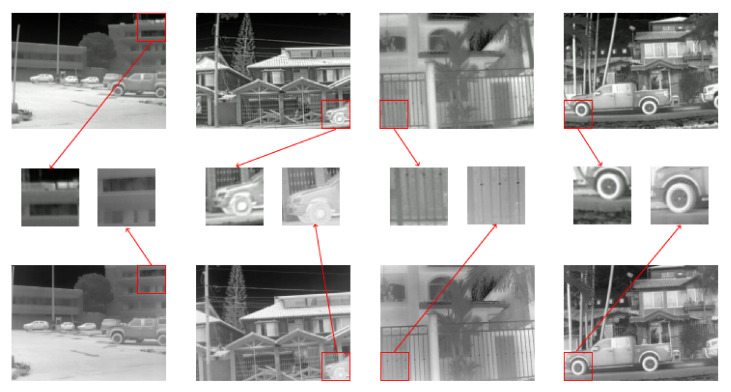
Examples of thermal images from [16]: (top) MR images from Axis Q2901-E (320 × 240), used in the current work as LR images; (bottom) HR images from FC-6320 FLIR (640 × 480) [16]; (middle) enlargements to show the misregistration between the images.
